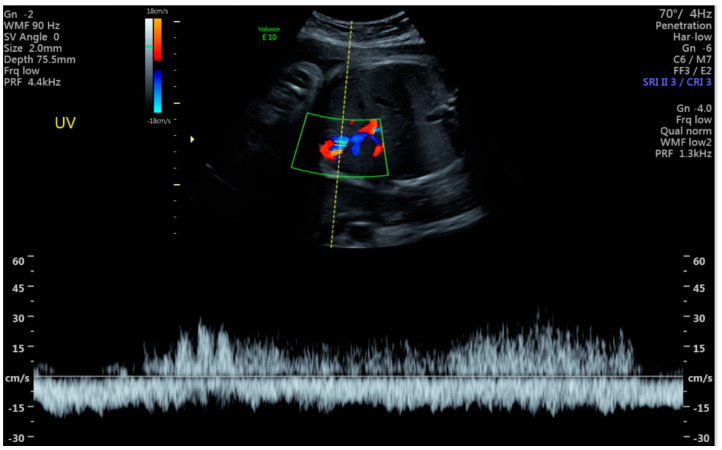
Ultrasound demonstrating an umbilical vein varix with color Doppler.
